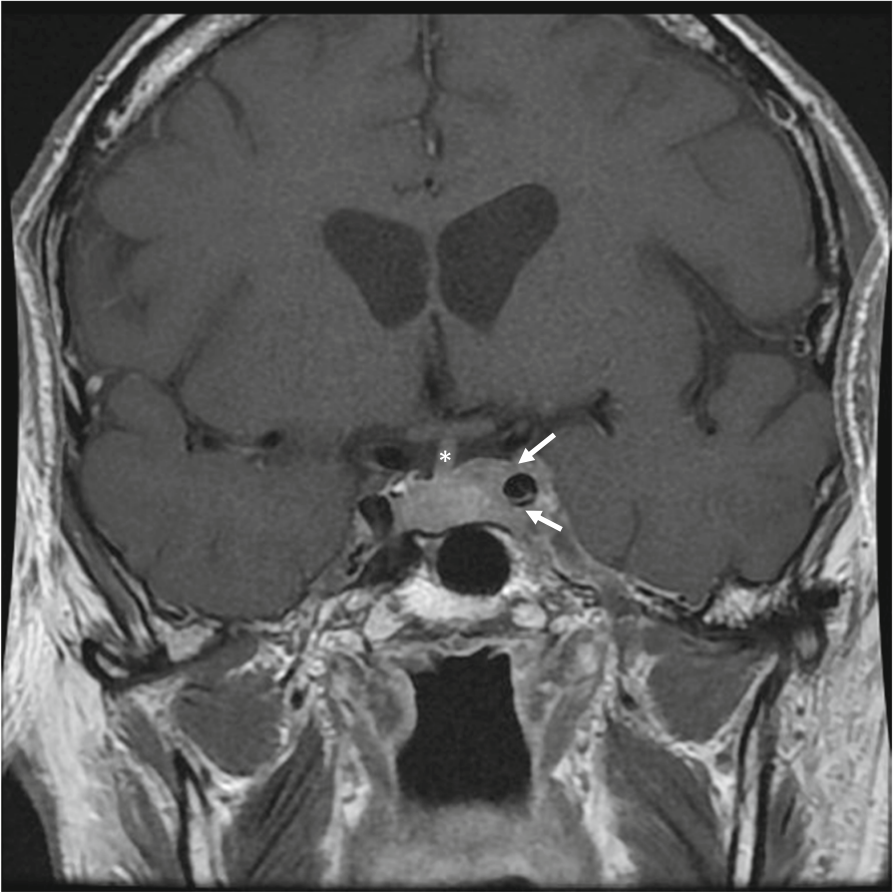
MRI scan of cavernous sinus mass. Coronal post-gadolium enhanced T1 MRI scan demonstrating soft tissue mass involving the left cavernous sinus and encasing the left internal carotid artery (arrows); note deviation of the hypothalamic-pituitary stalk to the right (*)
